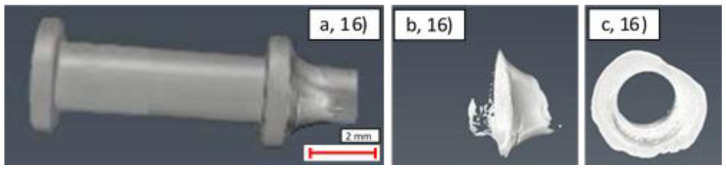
CT images of uncoated sample (16): (a) side view of gland exterior; (b) side view of interior solder; (c) axial view of interior solder.
